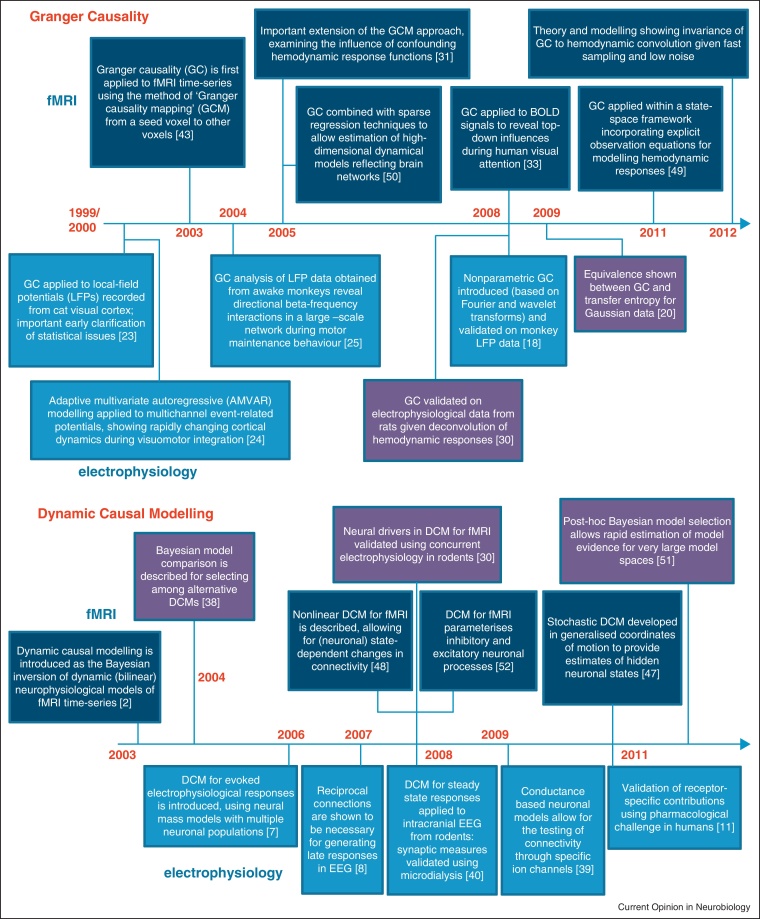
A timeline of recent advances in Granger causality (top panel) and dynamic causal modelling (bottom panel). Entries above the time lines pertain to functional magnetic resonance imaging (MRI) and those below the lines report specific developments for electrophysiology.
